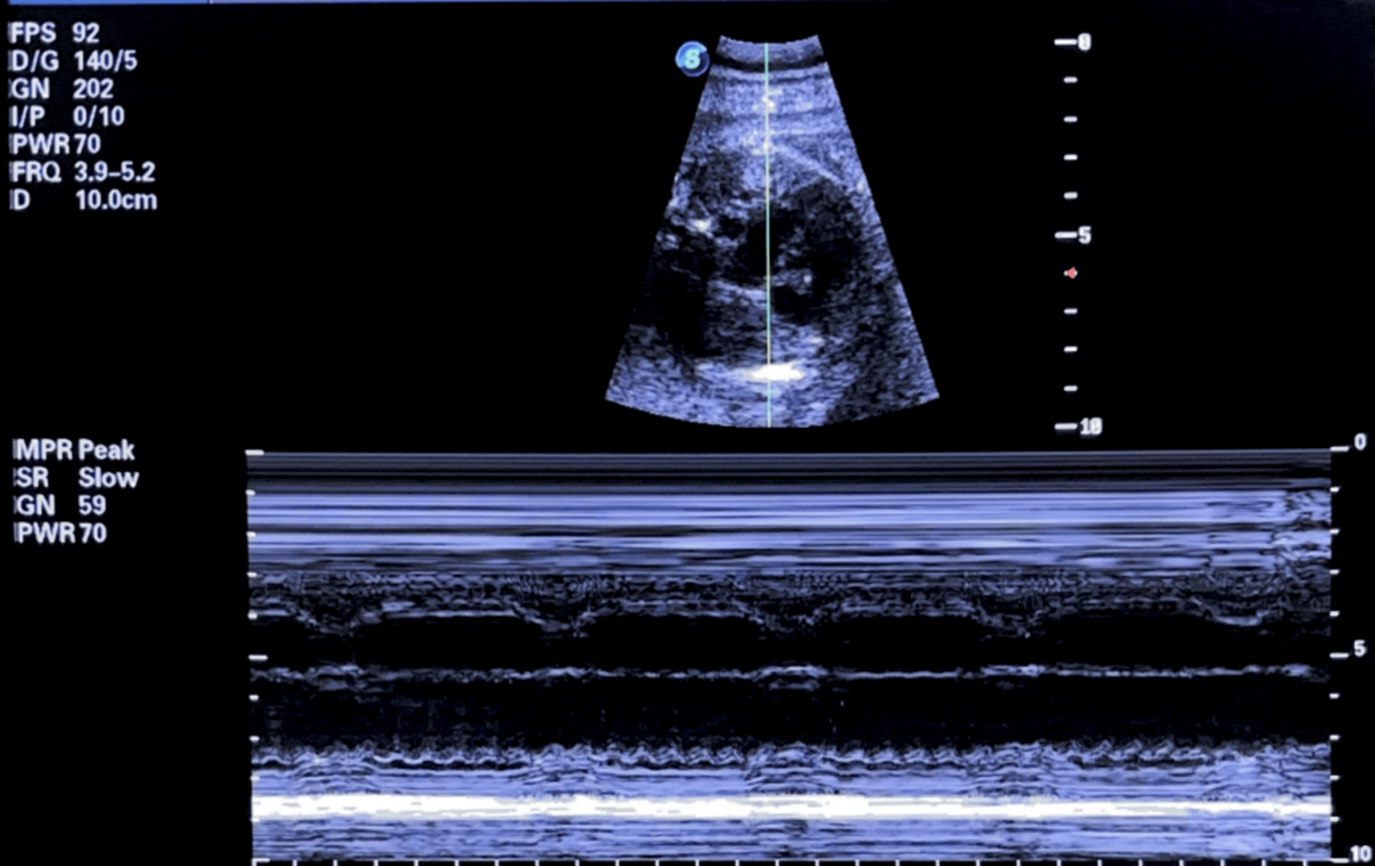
Fetal echocardiography in M-mode showing complete atrioventricular block Fetal M-mode echocardiography at 29 weeks' gestation showing complete atrioventricular block with a ventricular rate of 59 bpm and complete AV dissociation.
